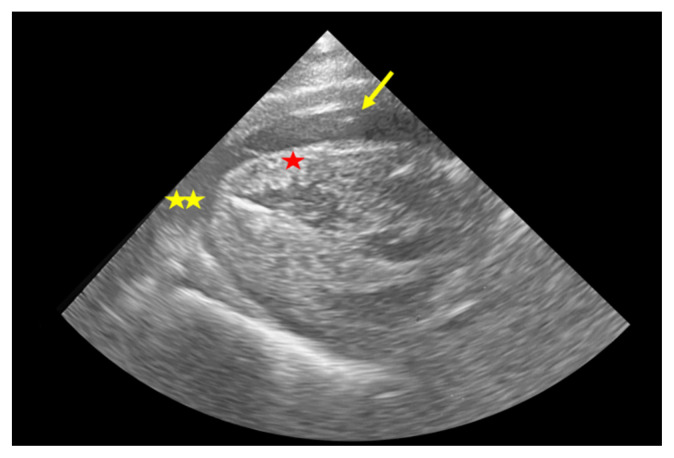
Parasternal long view of model effusion The myocardium (red star) and pericardial effusion (yellow stars) are easily visualized. Here a needle tip (arrow) can be seen aspirating the anterior pocket.
